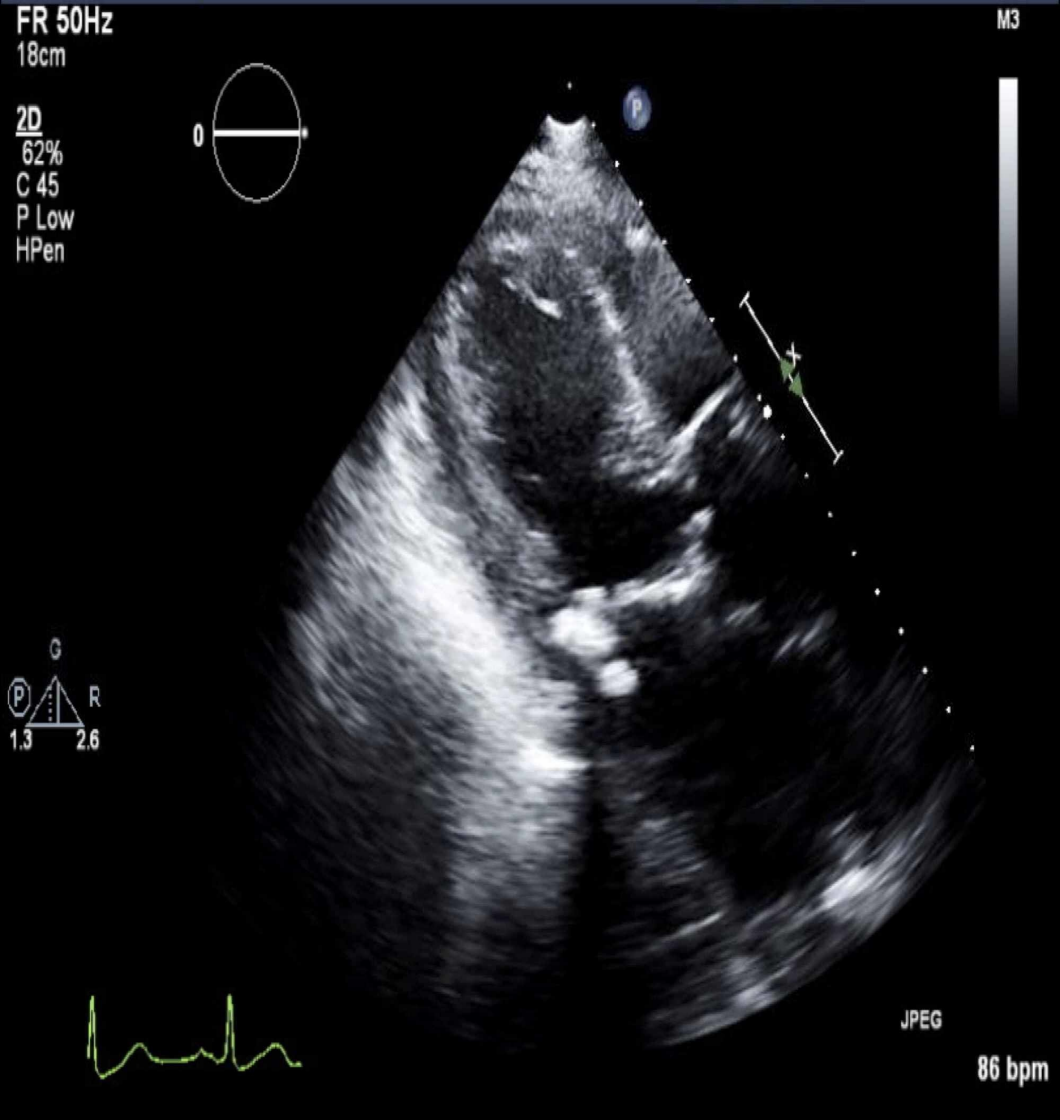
TEE with EF of 25% and a new anterior wall motion abnormality TEE: transesophageal echocardiography; EF: ejection fraction
